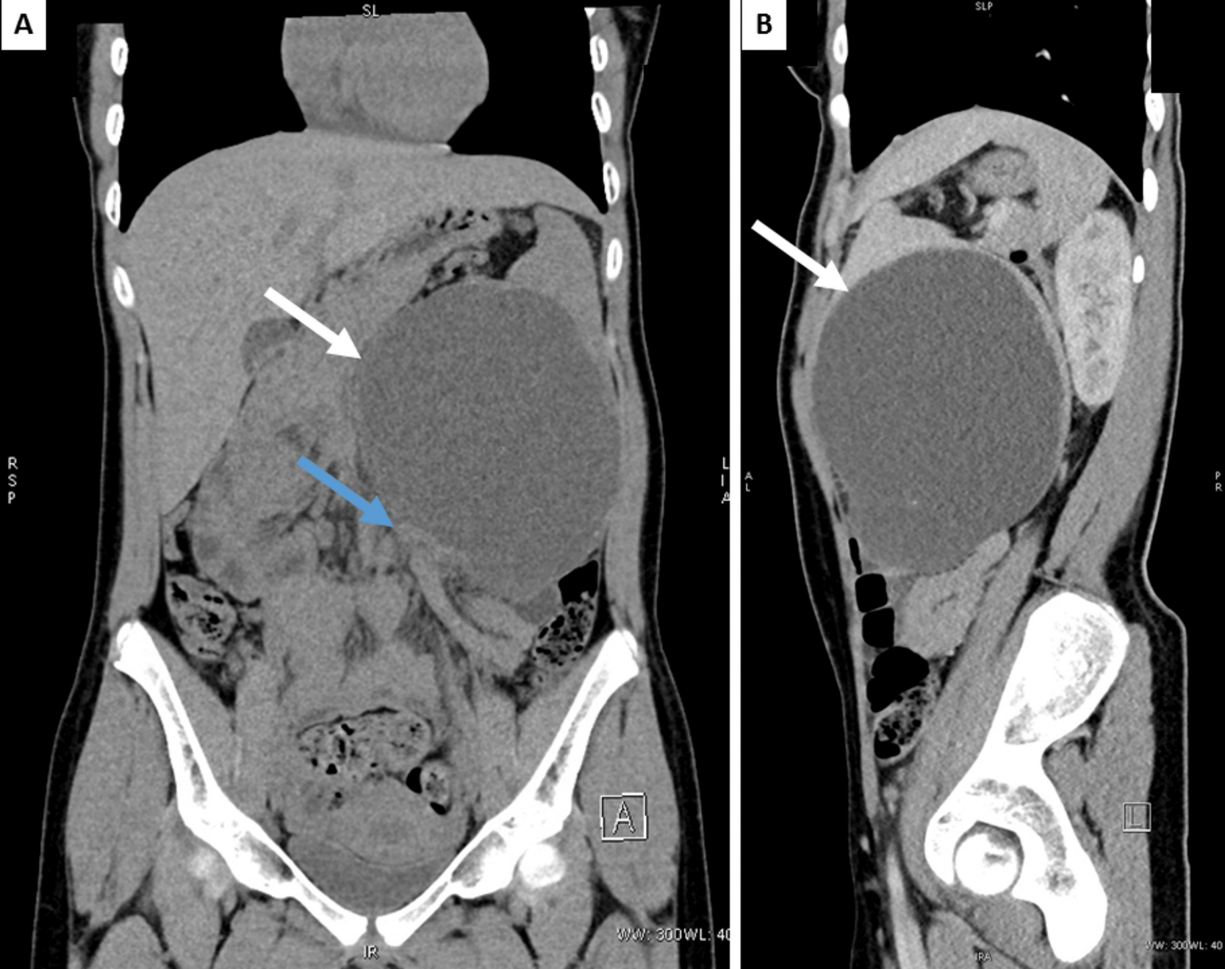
Abdominal computed tomography image A: Coronal view with a cystic lesion of the spleen (arrow) and renal compression (blue arrow); B: sagittal view with a smooth-walled cyst in the lower part of the spleen (arrow).
